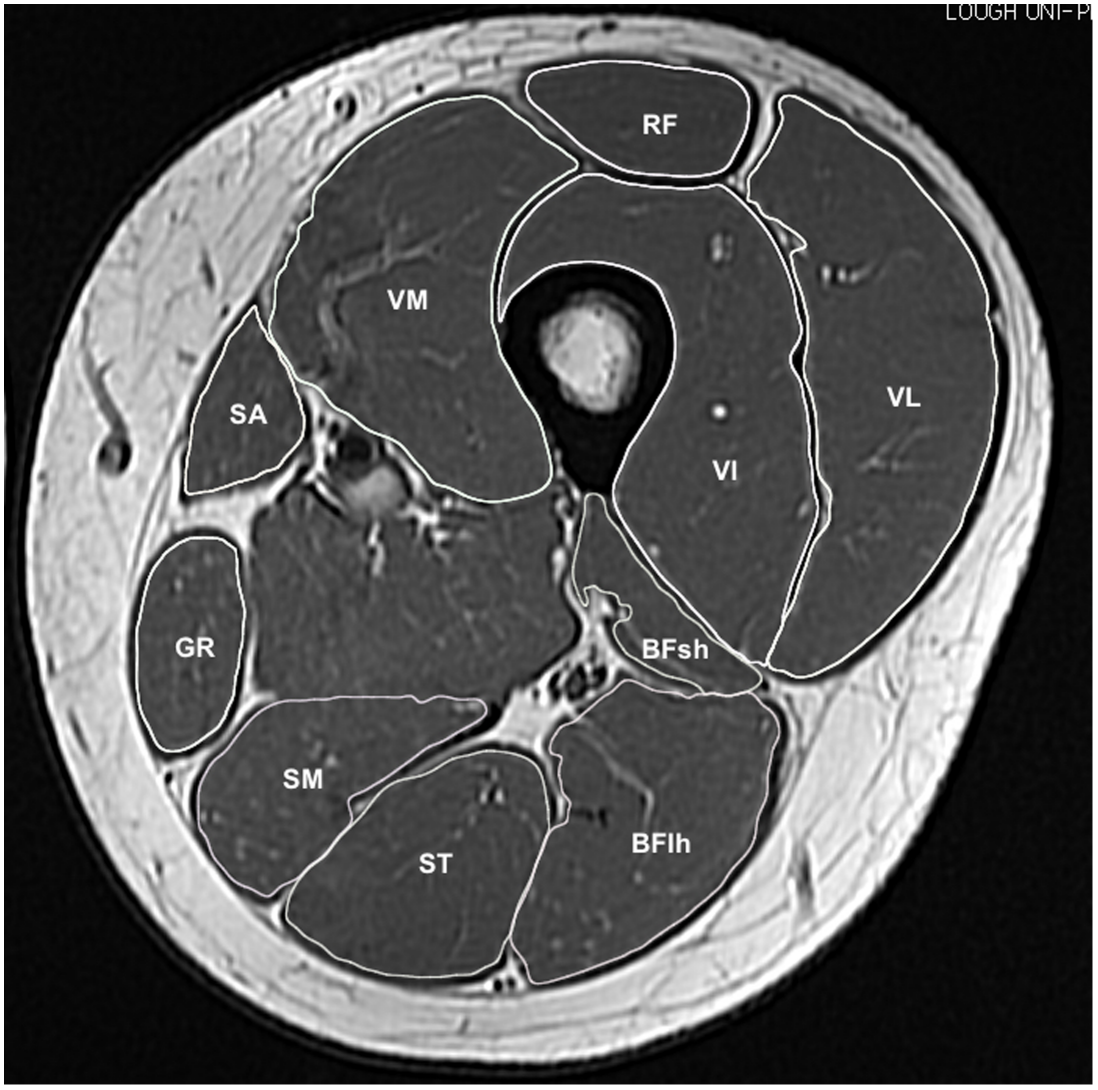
Example of MRI slice mid-thigh with the knee extensors and flexors manually segmented.
